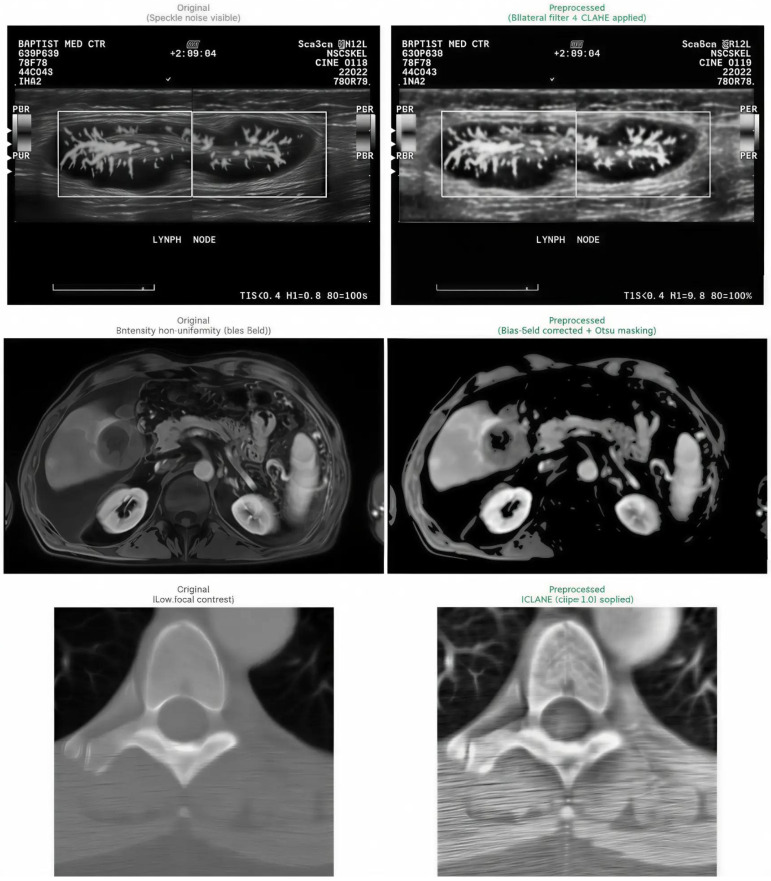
Representative qualitative examples of modality-specific preprocessing. Each row shows the original and processed image for ultrasound (bilateral filter + CLAHE), MRI (N4 bias-field correction + Otsu masking), and mammography (CLAHE). These examples provide qualitative evidence that the preprocessing protocol produces the intended modality-specific effects.
